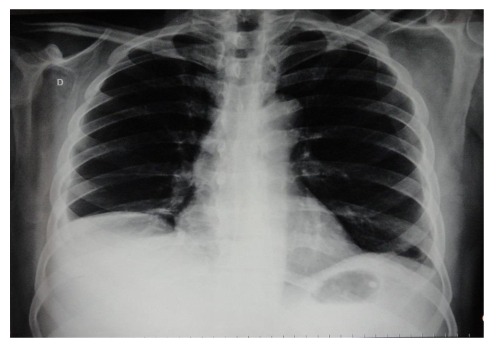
A chest X-ray showing moderate, right-sided pleural effusion.
